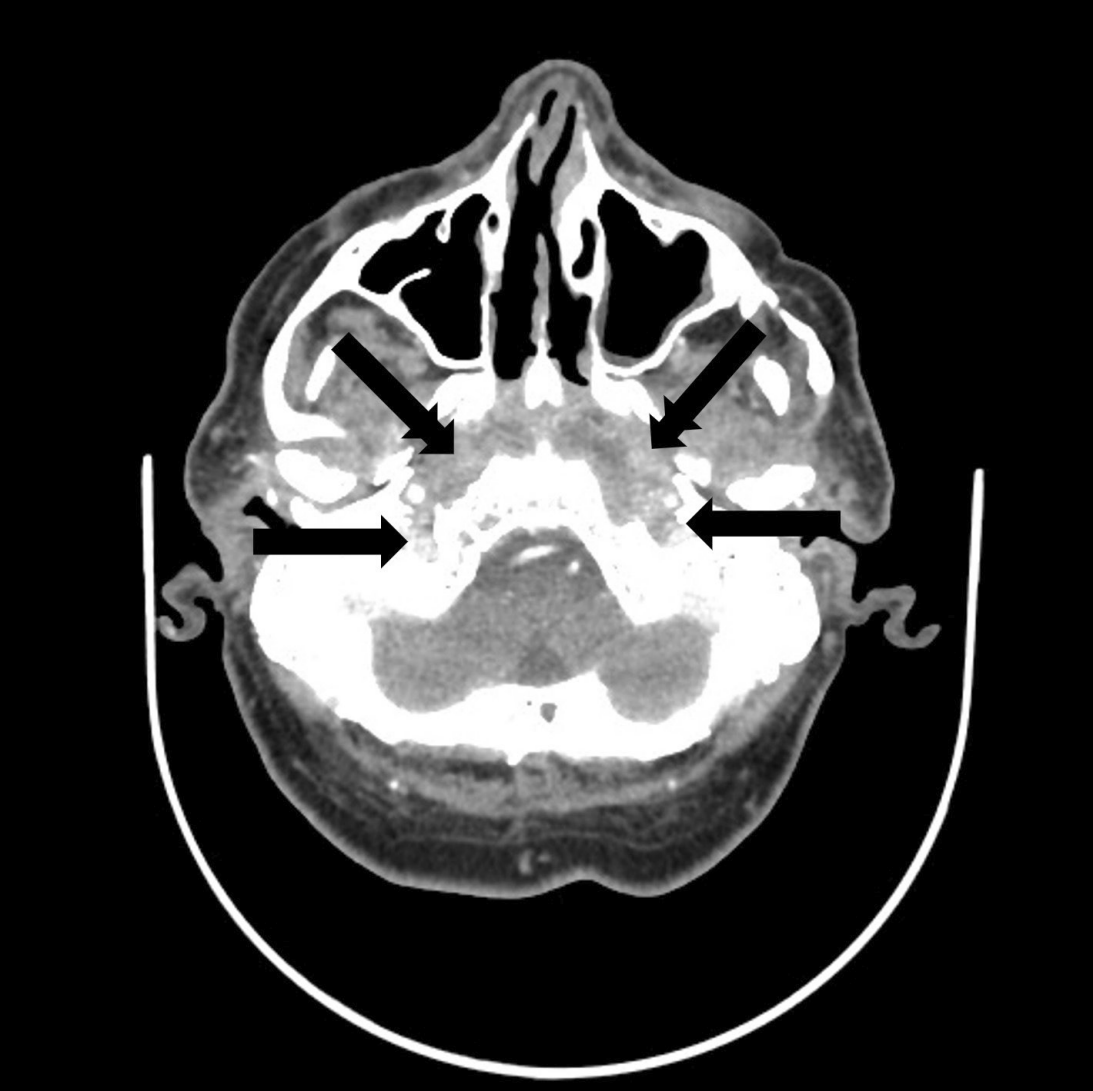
Axial CT scan showing bilateral partial erosion of the bony boundaries of the middle skull base (simple arrows) with moderate soft tissue contrast enhancement (double arrows).
